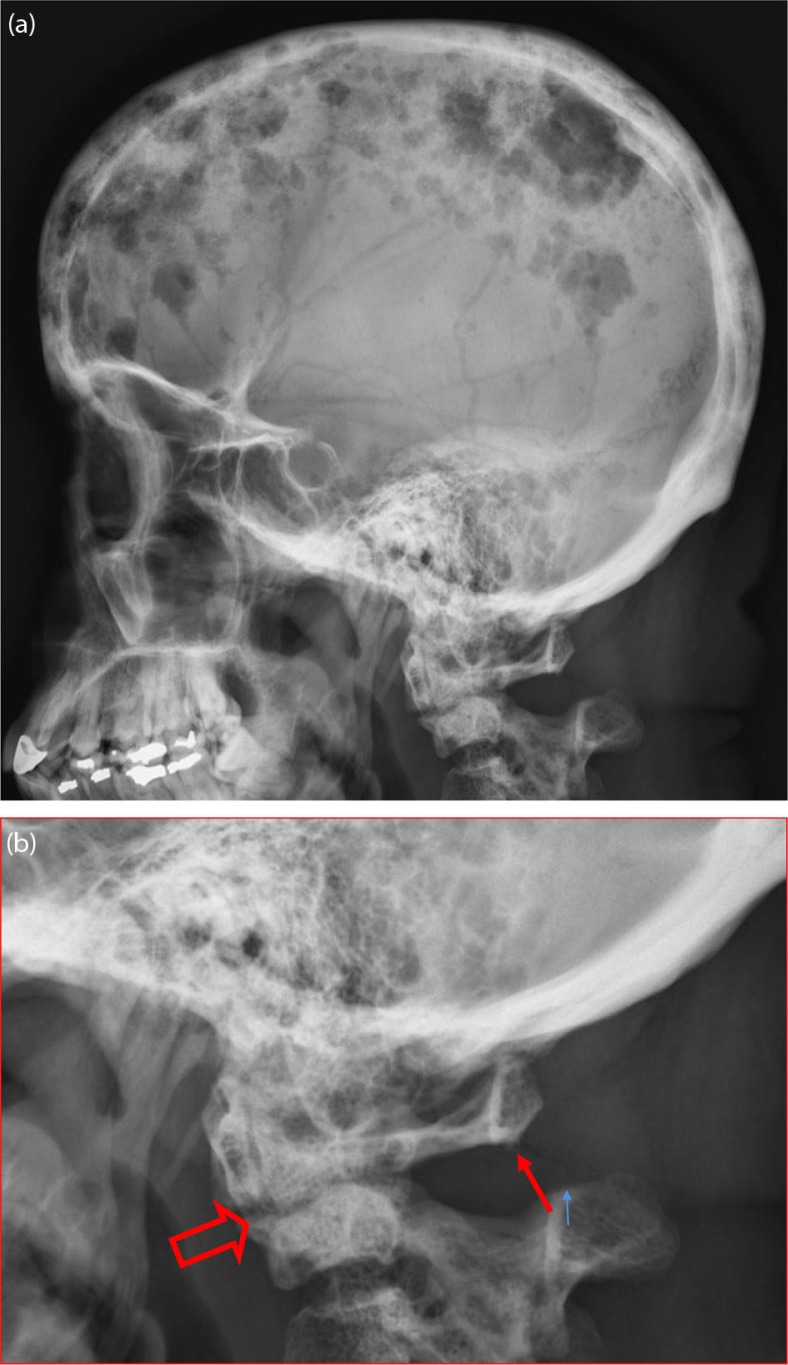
A lateral skull radiograph (a) shows numerous lytic lesions classic for myeloma. More subtle, at the craniocervical junction (b), there is a destructive lesion of C2 resulting in compression (open arrow), abnormal flexion (small arrow), and translation, and potential instability.
